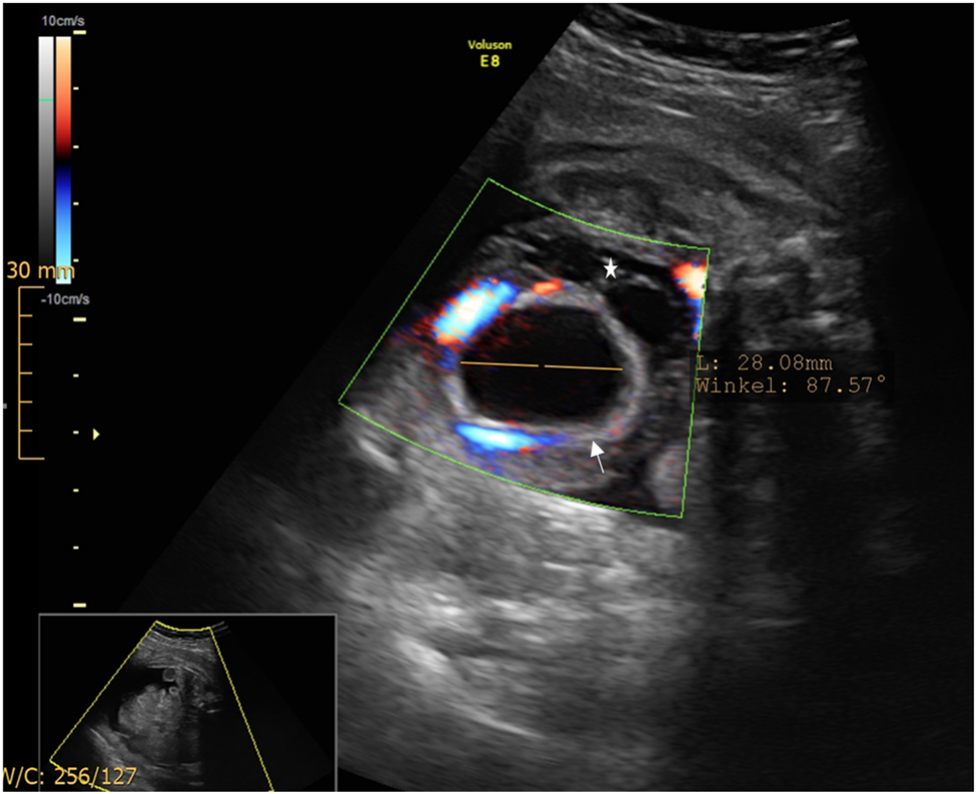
Ultrasound showing a decompressed fetal bladder (28 mm) with thickened walls (↑) and prevesical hematoma (*).
